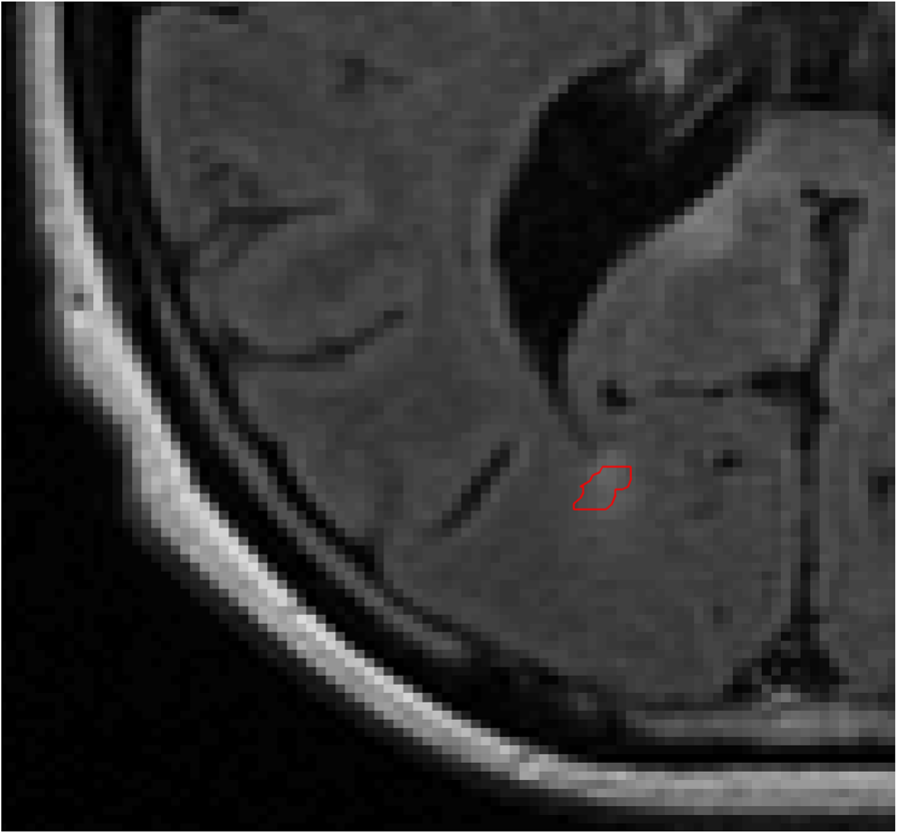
Flair MRI, with a hand-drawn ROI of a lesion shown in lower left.
